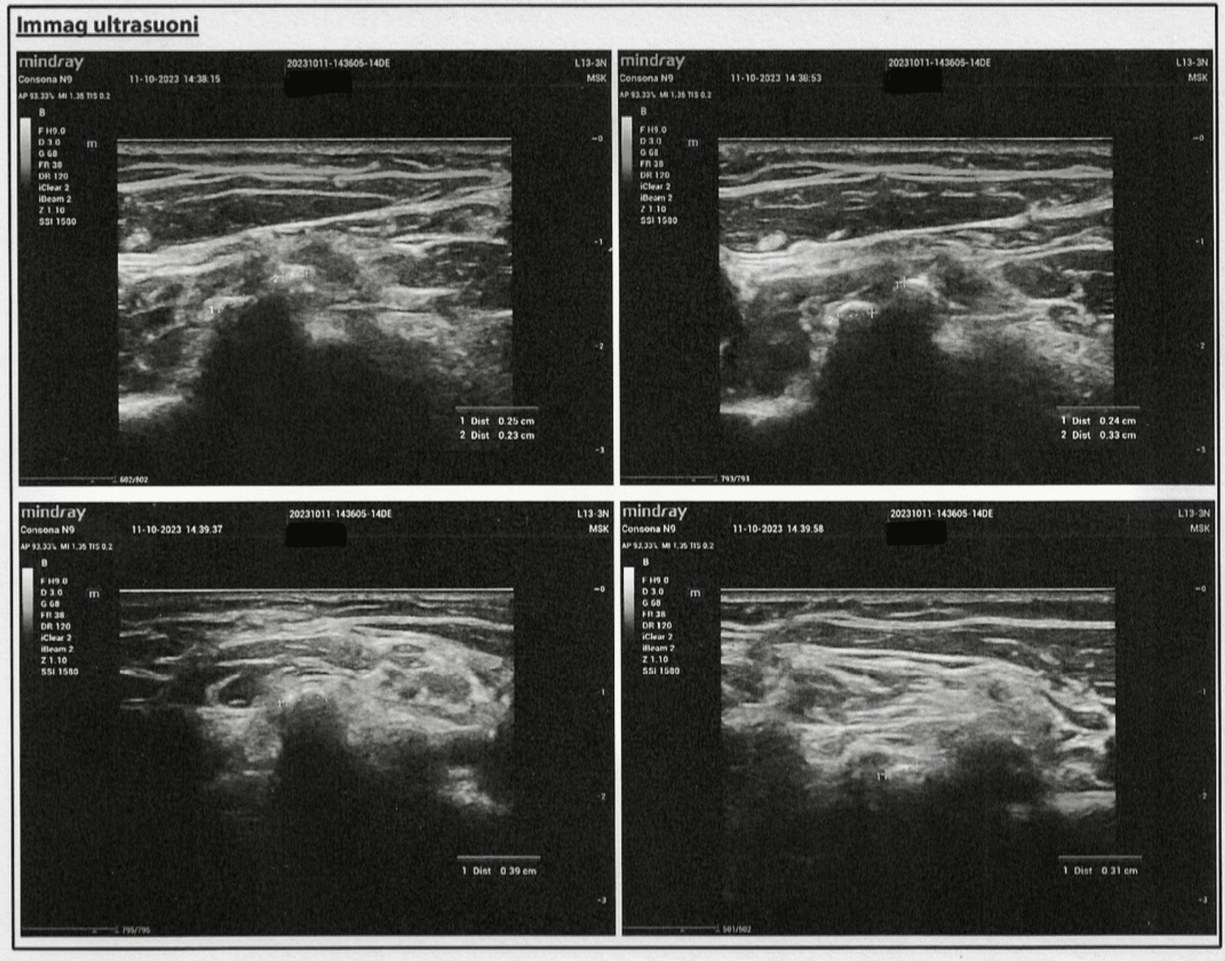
Ultrasound imaging of multiple small calcified deposits within the muscle belly of the sternocleidomastoid on both sides.
